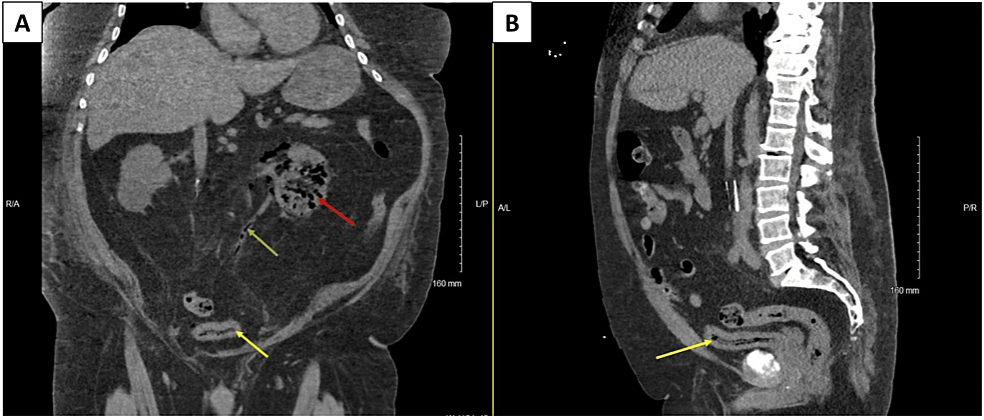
Non-contrast enhanced CT of the abdomen and pelvis Panel A: Coronal view showing gas within the left renal parenchyma (red arrow), wall of the left ureter (green arrow), and wall of the urinary bladder (yellow arrow). Panel B: Sagittal view showing gas within the urinary bladder (yellow arrow).
